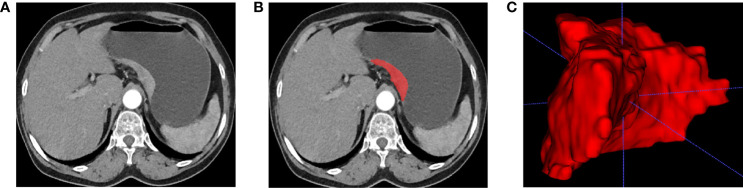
Tumor segmentation. (A) Enhanced CT arterial phase images show local gastric wall thickening and enhancement. (B) Manual segmentation. (C) 3D reconstruction of tumor.
